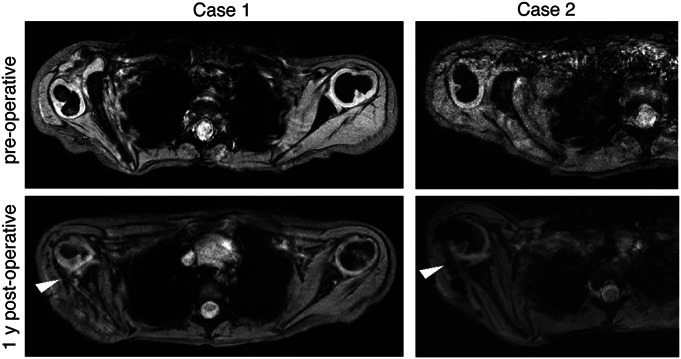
Axial magnetic resonance images of Waters V (Case 1) and Waters IV (Case 2) glenohumeral dysplasia cases that underwent glenoid anteversion osteotomy and soft tissue rebalancing. Transferred latissimus dorsi tendons with signal artefacts due to nonabsorbable sutures are marked by white arrowheads.
